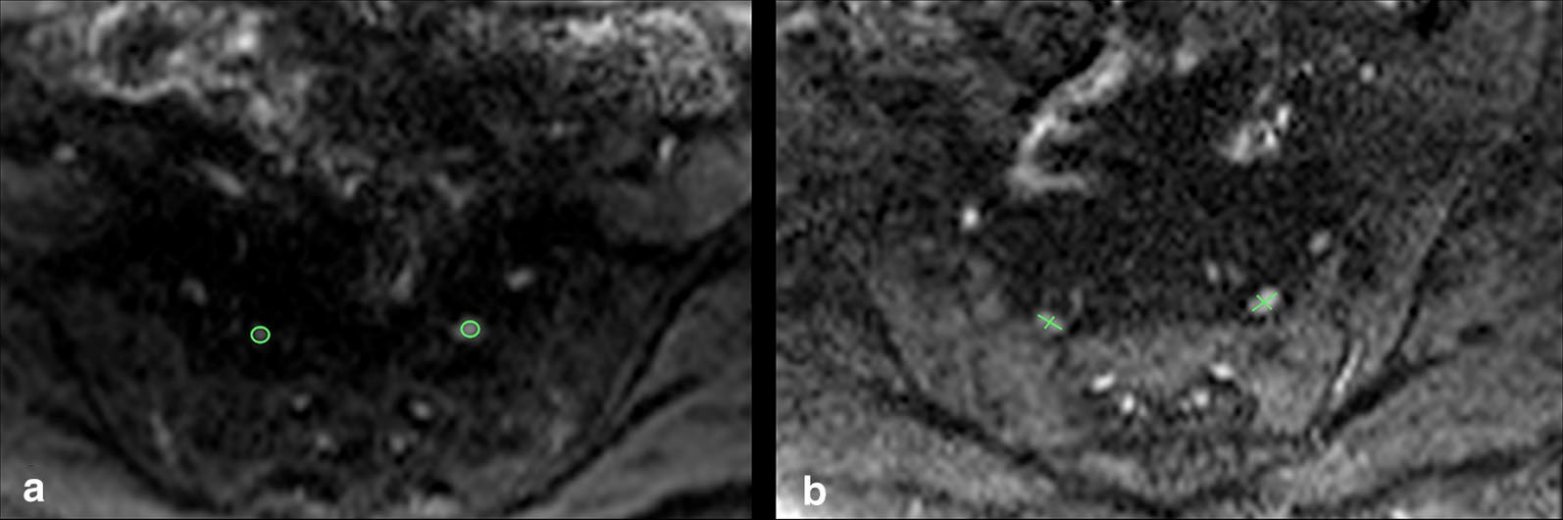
DWI-RESOLVE images on two different patients demonstrating typical nerve region of interest (ROI) placements (a) and dimensional calipers (b) for quantitative measurements
